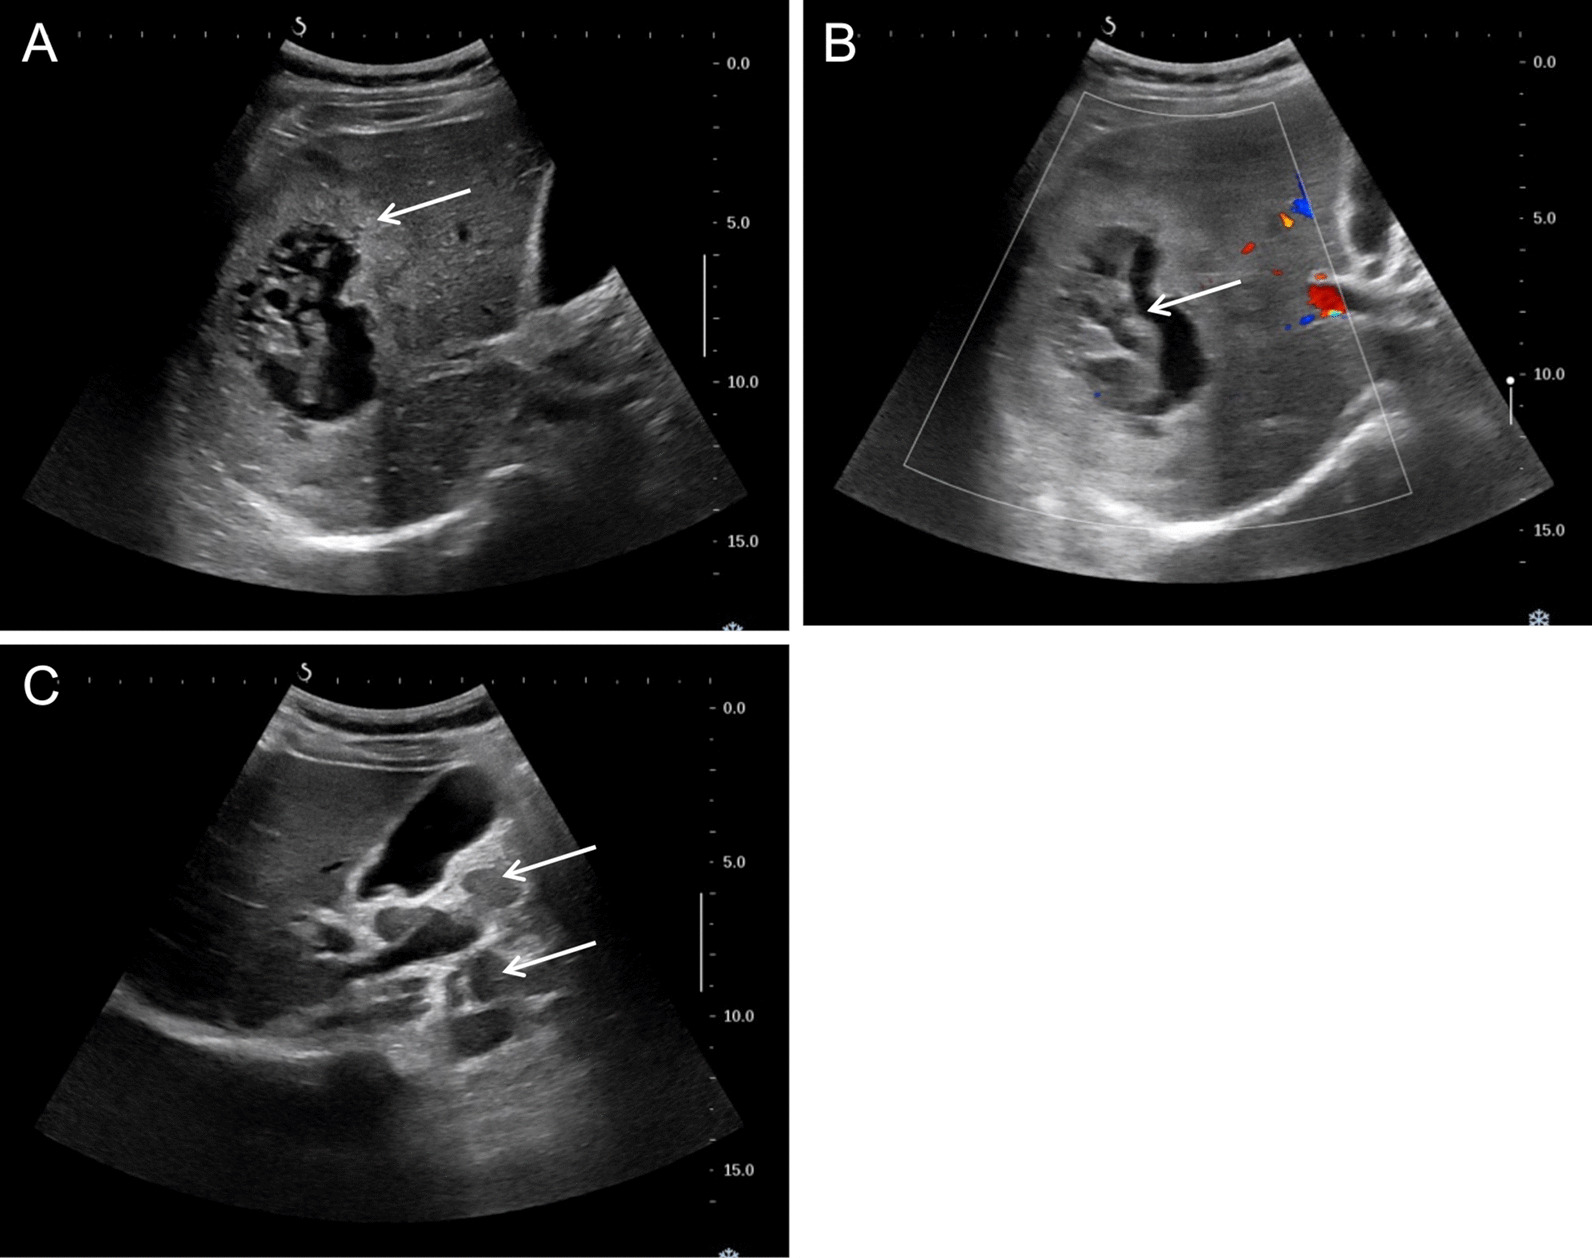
Ultrasonography images of case 1
